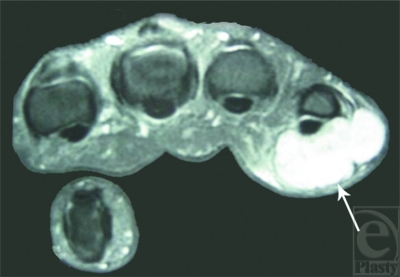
Preoperative magnetic resonance imaging (MRI). Gadolinium-enhanced, T1-weighted MRI shows a 2.3 × 2.3 × 1.3-cm lobulated, sharply circumscribed lesion (arrow) lying on the volar aspect of the little finger proximal phalanx and metacarpophalangeal joint, without cortex or tendon invasion.
